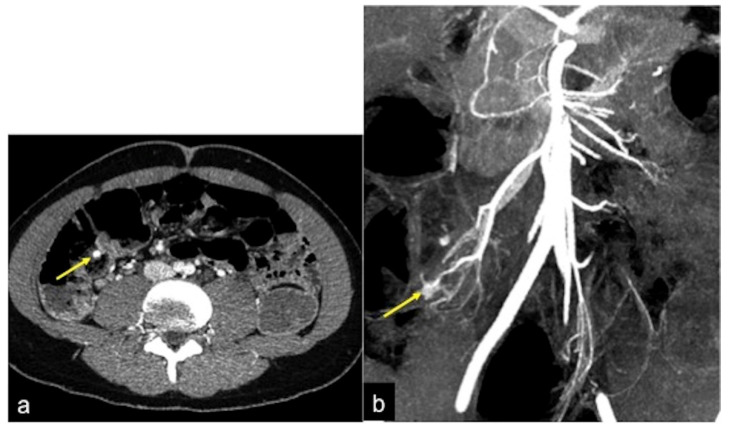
CTA study of a haemorrhagic spot in the ascendant colon. A vascular nidus (arrow) is detected in the arterial phase (a); the MIP post-processing with MPR coronal reconstruction facilitates the identification of the vascular malformation (arrow) in order to better define the vascular anatomy for the treatment approach (b).
